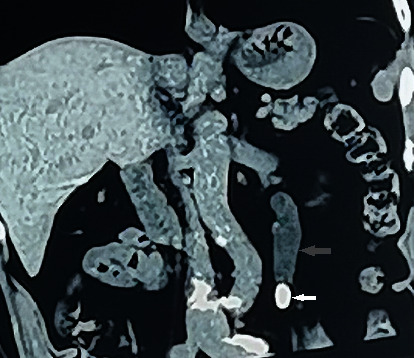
CT image in 2013 shows left ureteral effusion (grey arrow), with calculus completely obstructing the ureter (white arrow; the low-density shadow around the calculus is considered as ureteral wall edema caused by the calculus).
